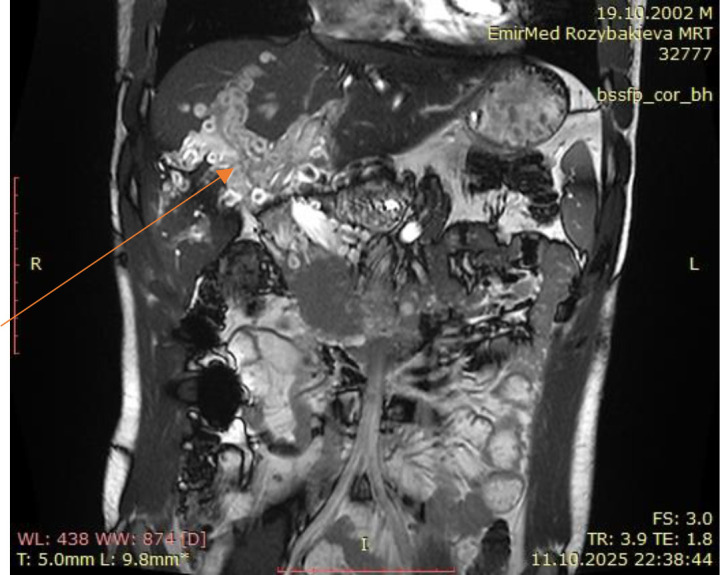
Targeted NF1 lesions in the liver originating from the celiac trunk in the patient on 3 Tesla MRI (5 mm slice thickness) in Fat Saturation (FS) regime.
